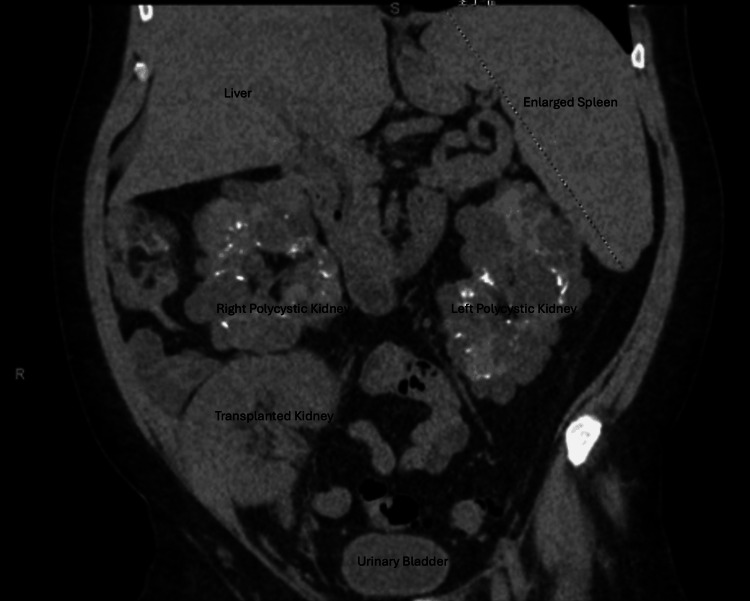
Coronal view of CT scan of the abdomen and pelvis. The image shows the transplanted kidney in the right lower quadrant. Enlarged native kidneys with numerous cysts, moderately enlarged spleen measuring 19 cm in maximal craniocaudal dimension.
